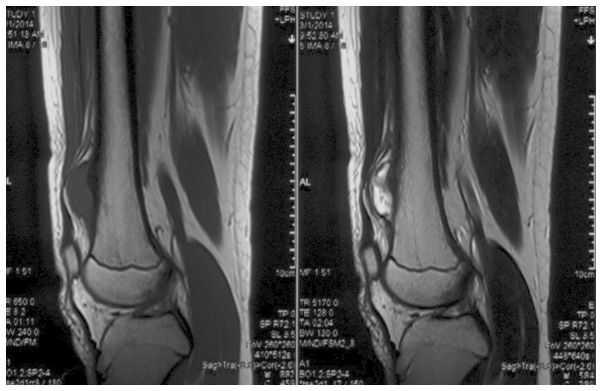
Magnetic resonance imaging revealed a lobular heterogeneous mass arising from the femur periosteum.
